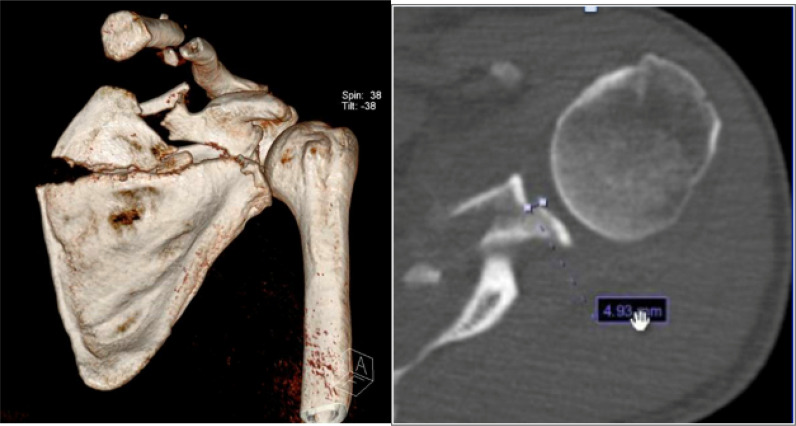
Three-dimensional reconstruction and axial computed tomography scan of a patient with left glenoid displaced fracture that was treated nonoperatively.
